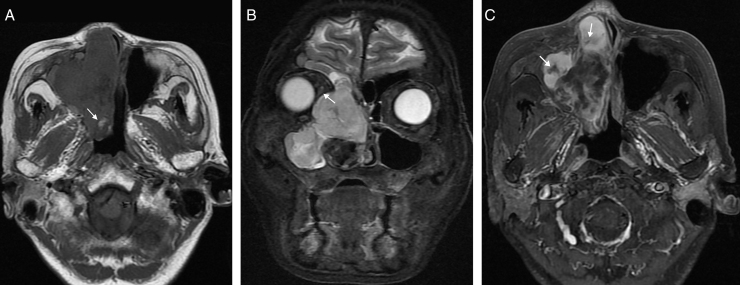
(A) Axial T1-weighted image shows lower-signal intensity and high-signal intensity. The surrounding structures are compressed. Hemorrhagic focus can be found in the mass (white arrow in A). (B) Coronal T2WI with fat suppression shows the lesion more obviously. The lesion involved the right ethmoidal sinus, right maxillary sinus, right frontal sinus and right nasal cavity. Medial rectus was compressed to the right side by the lesion (white arrow in B). (C) Contrast-enhanced T2-weighted image with fat-suppression shows heterogeneous enhancement of the soft tissue mass and striped dis-enhanced region (white arrow in C).
